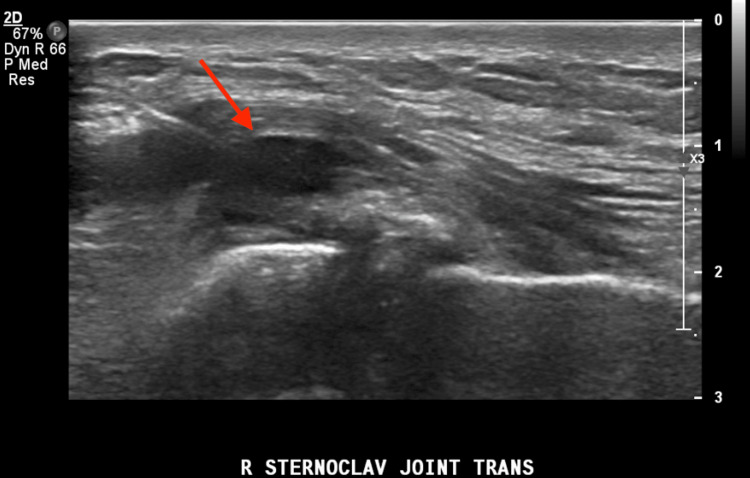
Ultrasound aspiration of the right sternoclavicular joint (red arrow)
